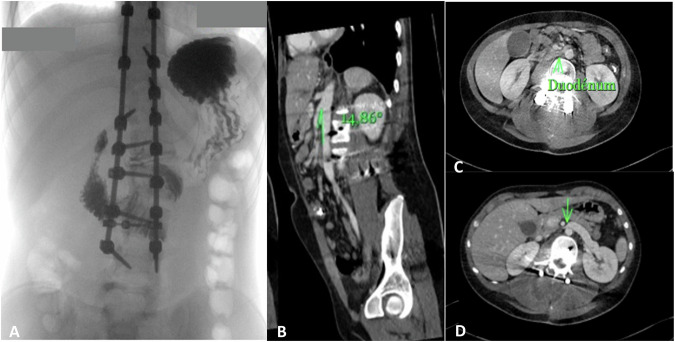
Imaging of the patient confirming superior mesenteric artery syndrome. A Upper gastrointestinal contrast showing transient stagnation of contrast in second duodenum. B sagittal view of abdominal CT scan revealing the reduction of the arto-mesentérique angle at 14° (normal > 25°). C axial view of abdominal CT scan revealing duodenal compression between the mesenteric (in front) and the aortic (behind) artery. D Axial view of abdominal CT scan revealing left renal vein compression and upstream expansion.
